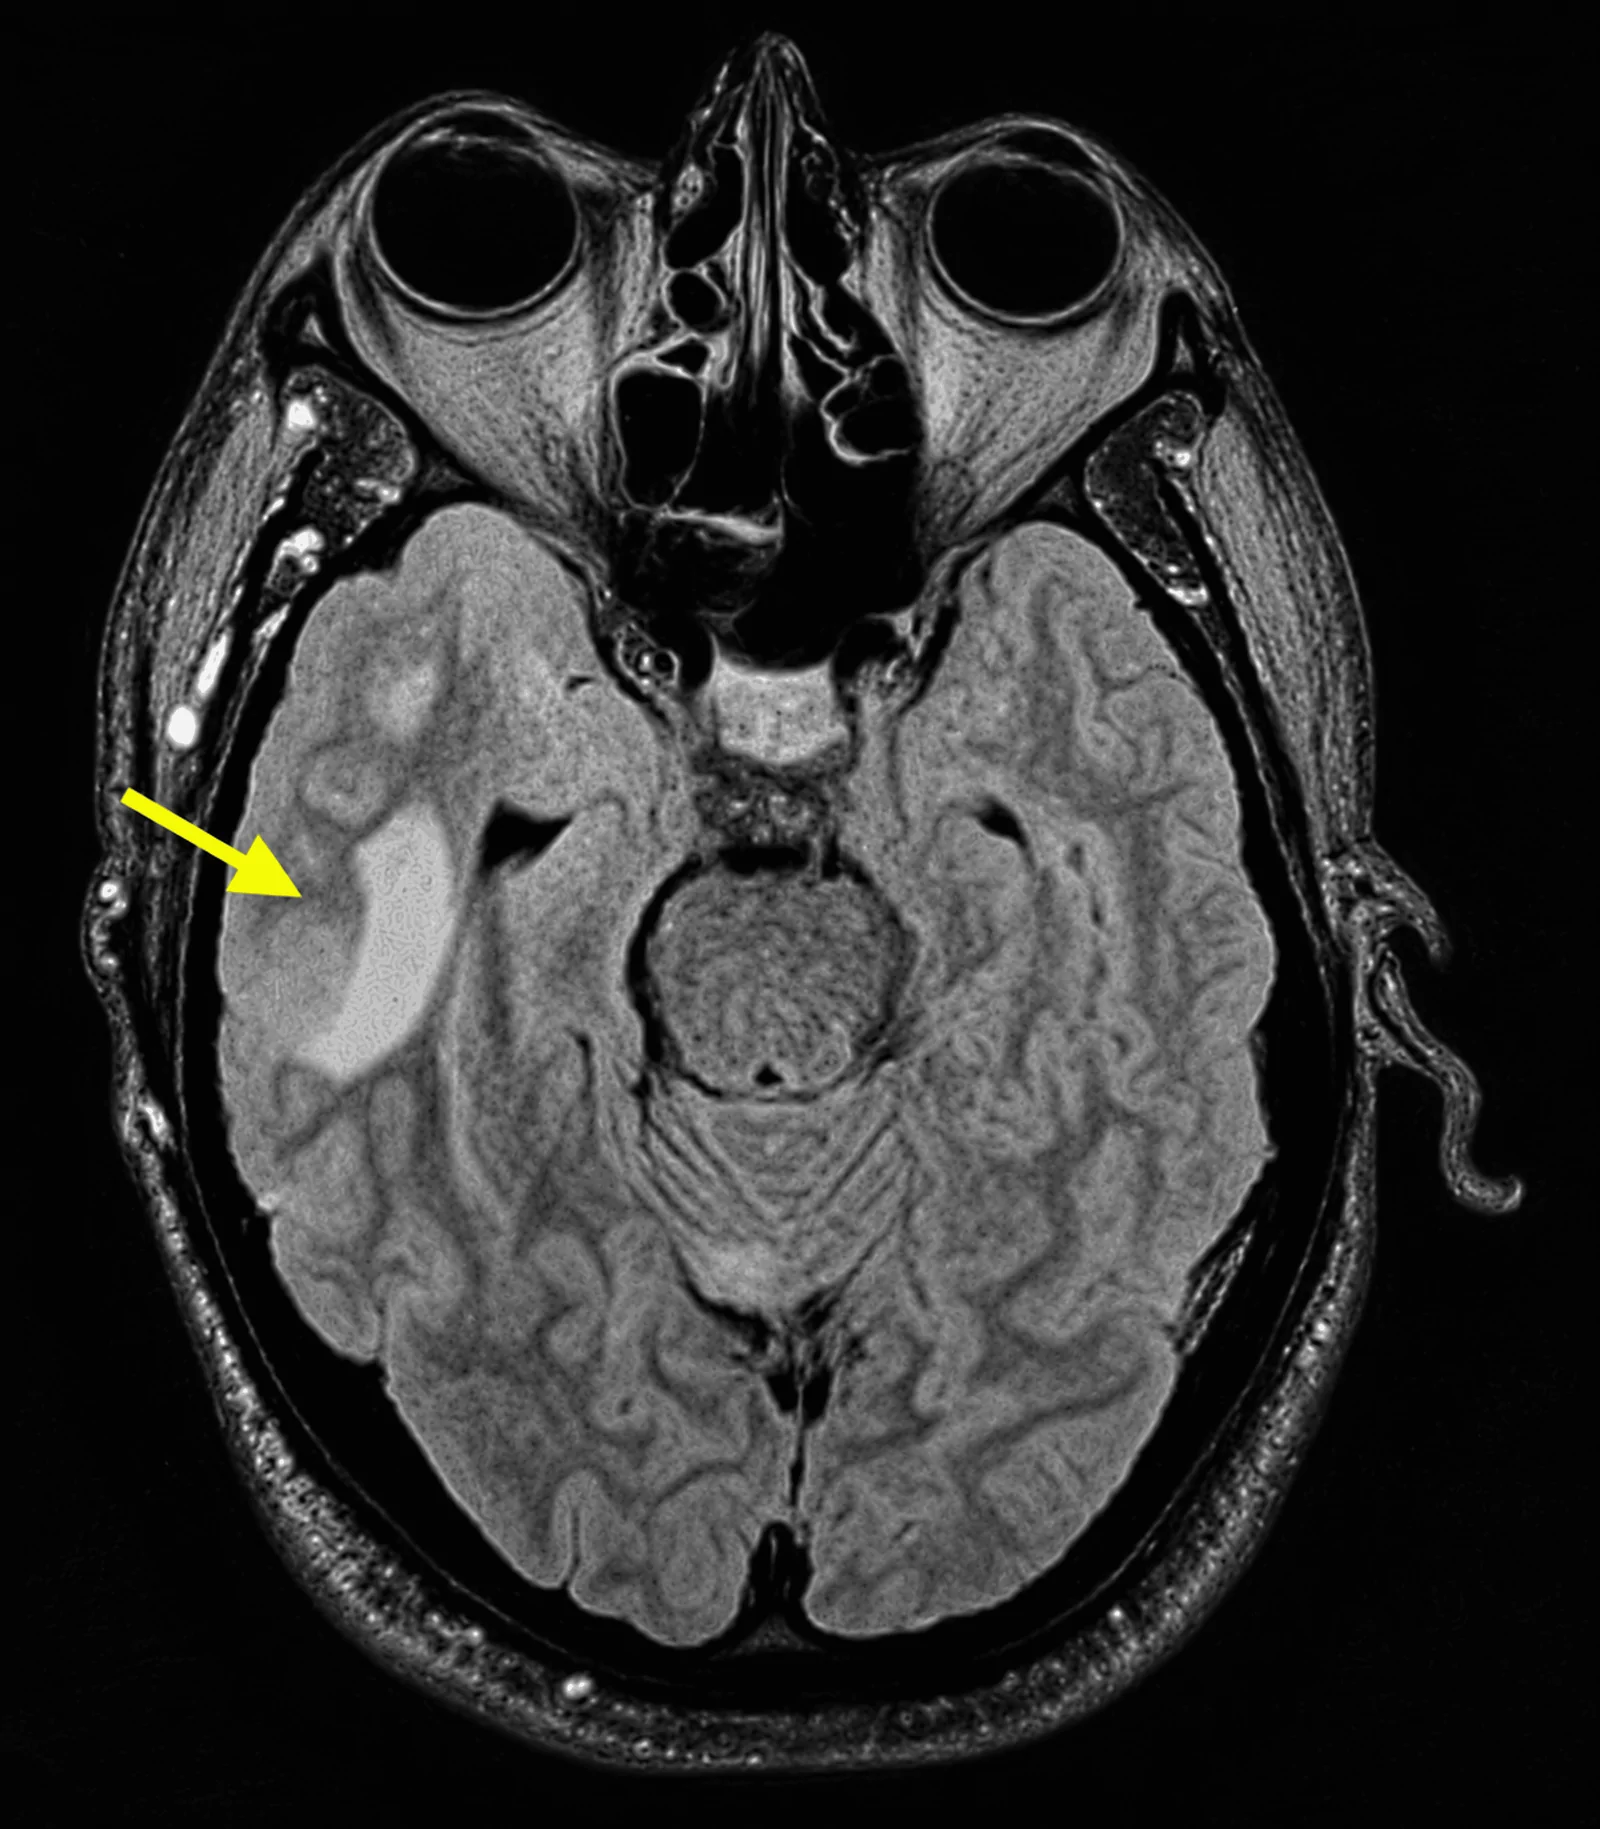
Axial FLAIR MRI showing right temporal cortical and subcortical hyperintensities consistent with infectious meningoencephalitis (yellow arrow) The FLAIR hyperintense abnormalities involve the right temporal cortex and adjacent subcortical white matter (yellow arrow), demonstrating the temporal distribution of the abnormalities associated with infectious meningoencephalitis. FLAIR: fluid-attenuated inversion recovery
